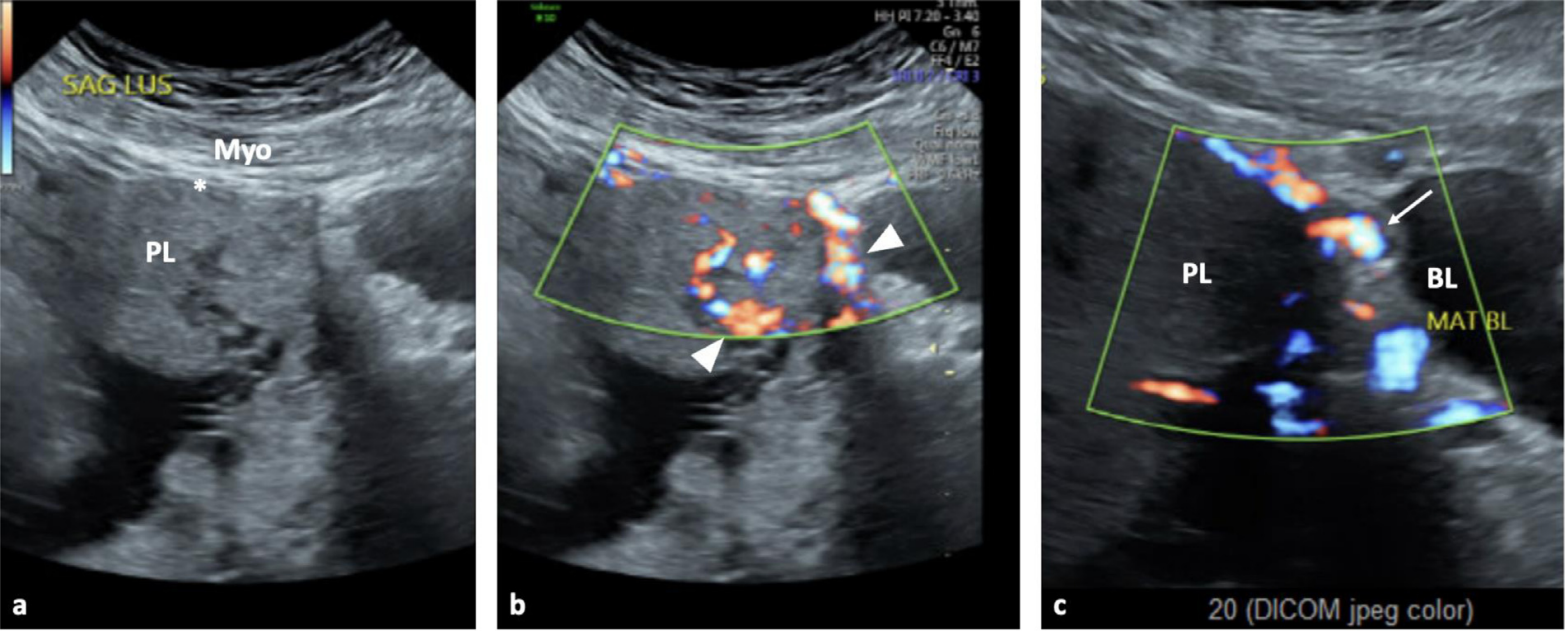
Grayscale (A) and color Doppler (B) transabdominal ultrasound images at the level of the lower uterine segment demonstrating loss of the retroplacental hypoechoic space (asterisk) and vascular lacunae (arrowhead) with placenta (PL) directly abutting the myometrium (Myo). Additional color Doppler ultrasound image of the pelvis (C) demonstrates increased vascularity with probable uterine wall invasion (arrows) adjacent to the bladder (BL).
